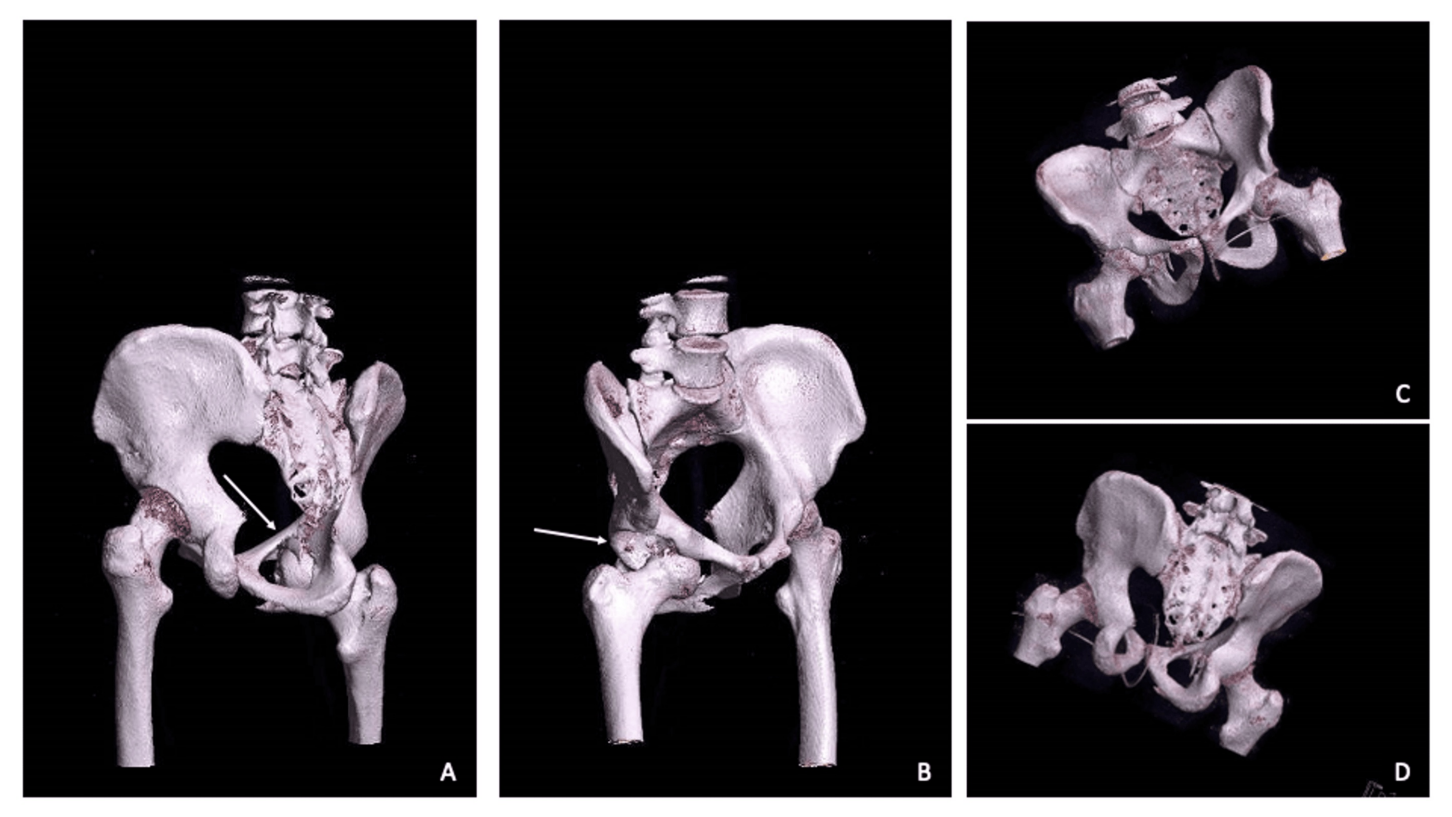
3D CT scans demonstrating a right obturator hip dislocation with concomitant fractures of the femoral head (arrow, A) and anterior acetabular wall (arrow, B), and after closed reduction (C, D)
